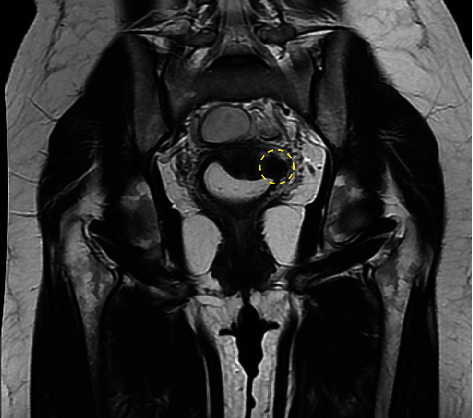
Coronal T2-weighted MRI. Tumor in the left paracolpium demarcated in yellow.
